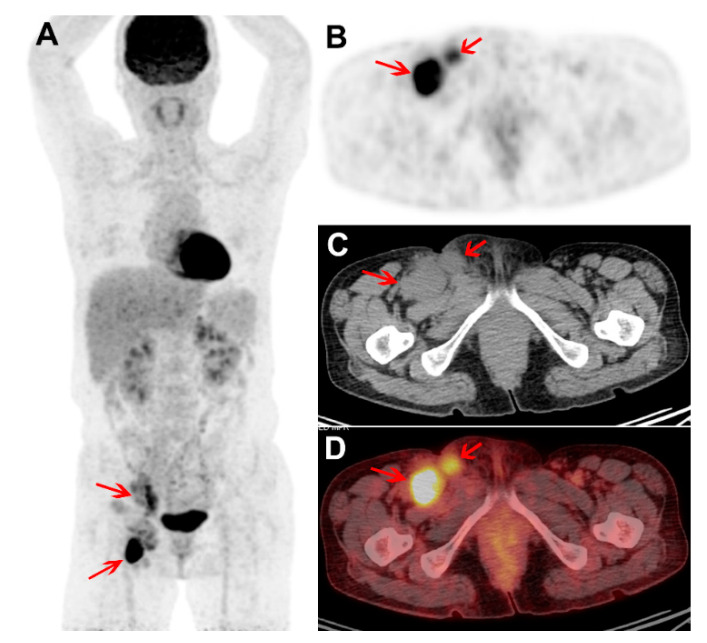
Treatment naïve FDG PET/CT images of a male with plasma cell subtype of multicentric CD (MCD). Maximum intensity projection PET (A) and trans-axial PET, CT, and fused PET/CT images (B–D) show intense FDG uptake in enlarged right external iliac and inguinal nodes (arrows; SUVmax 10.2 and T/L ratio 4.9). The FDG uptake intensity is high, but only two nodal stations were involved. The hemoglobin, platelet, albumin, and hs-CRP levels were within normal range. The patient received chemotherapy and siltuximab. Four years after the PET/CT study, the patient currently remains symptom free.
